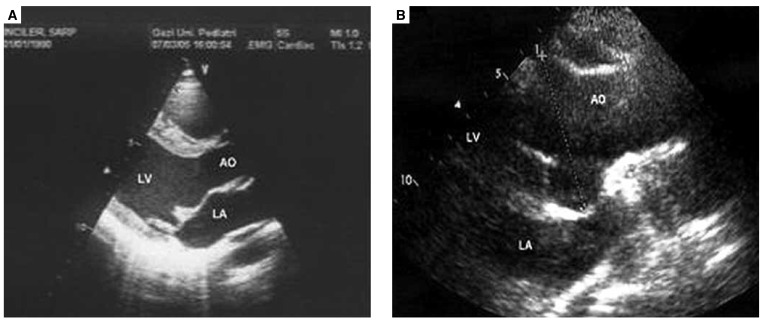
Mitral valve prolapse (A), and dilatation of the aortic root (B) in cross-sectional echocardiographic examination. AO: aorta, LA: left atrium, LV : left ventricle.
